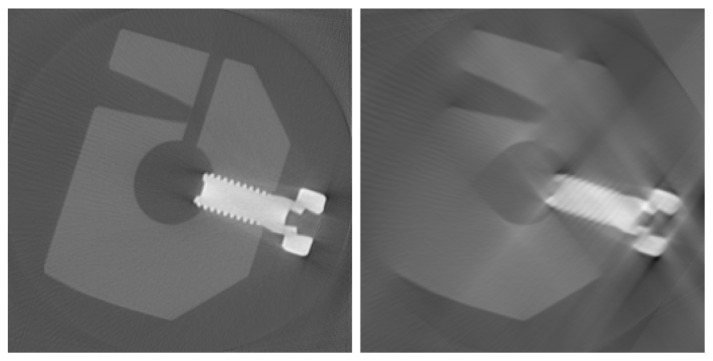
Cross sections through the reconstructed volumes from series of measured cone beam X-ray images spanning 360° (left image) and 100° (right image). They show an aluminium object with steel screw. The reconstructed density is proportional to the grey.
